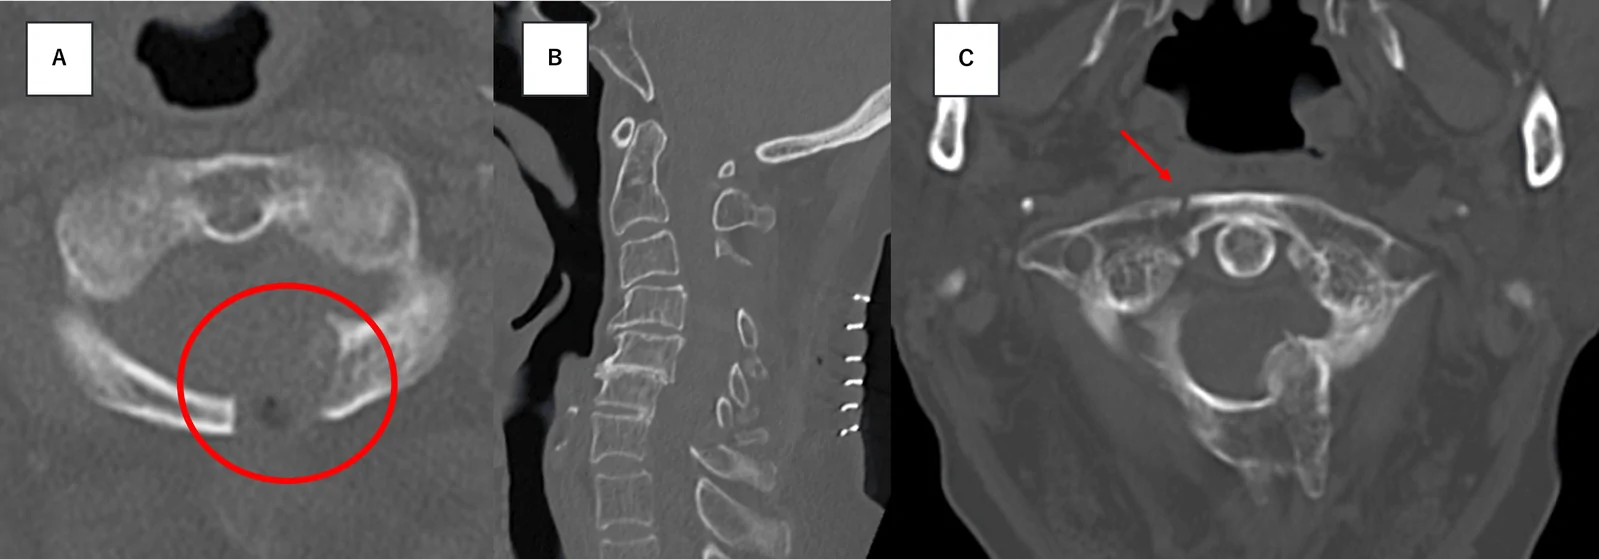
Preoperative and early postoperative imaging findings (A) Axial CT image following left unilateral C1 laminectomy (10.49 mm resection width). (B) Sagittal CT image after surgery. (C) CT obtained on postoperative day 6 demonstrating right anterior arch fracture of C1 CT: computed tomography
